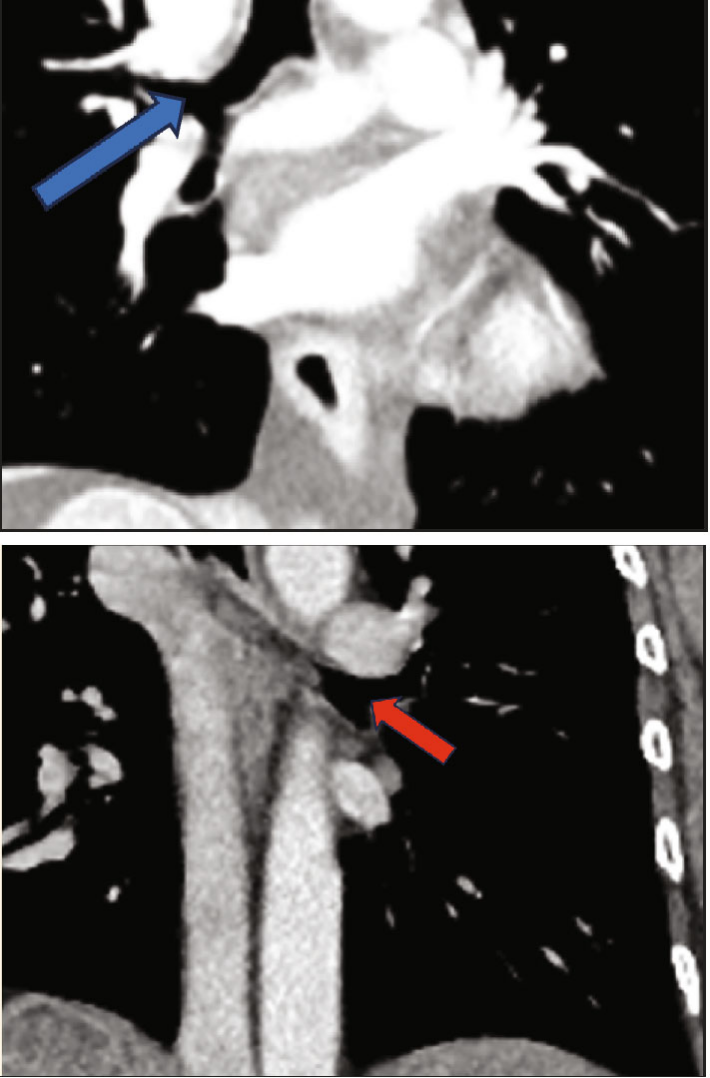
Coronal CT demonstrates normal eparterial right upper lobe bronchus (blue arrow) and hyparterial left upper lobe bronchus (red arrow).
